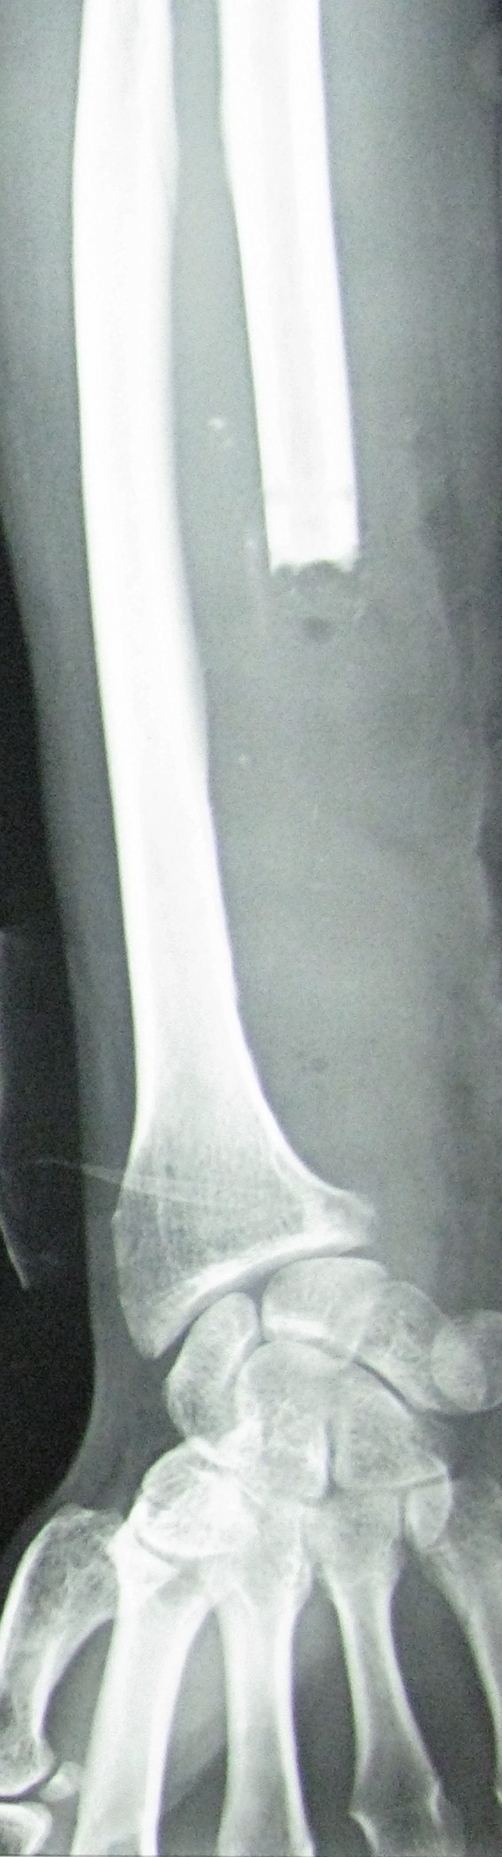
AP radiograph of Case #1 at 3 years of follow up.
